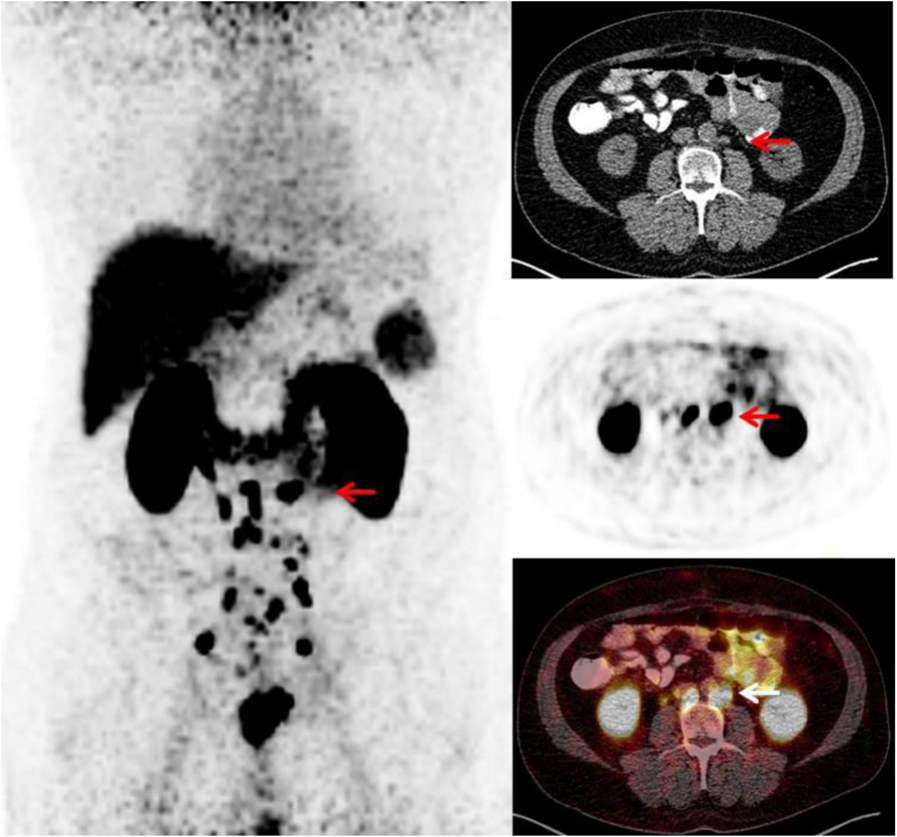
A 62-year-old patient with persisting PSA level (0.7 ng/ml) after radical prostatectomy. Initial PSA level 9.24 ng/ml. High-risk (G3) prostate cancer pT3aN1. ^68^Ga-PSMA PET/CT detected multiple small pelvic and retroperitoneal (*arrow*) LNMs. The intended treatment was changed to ADT
